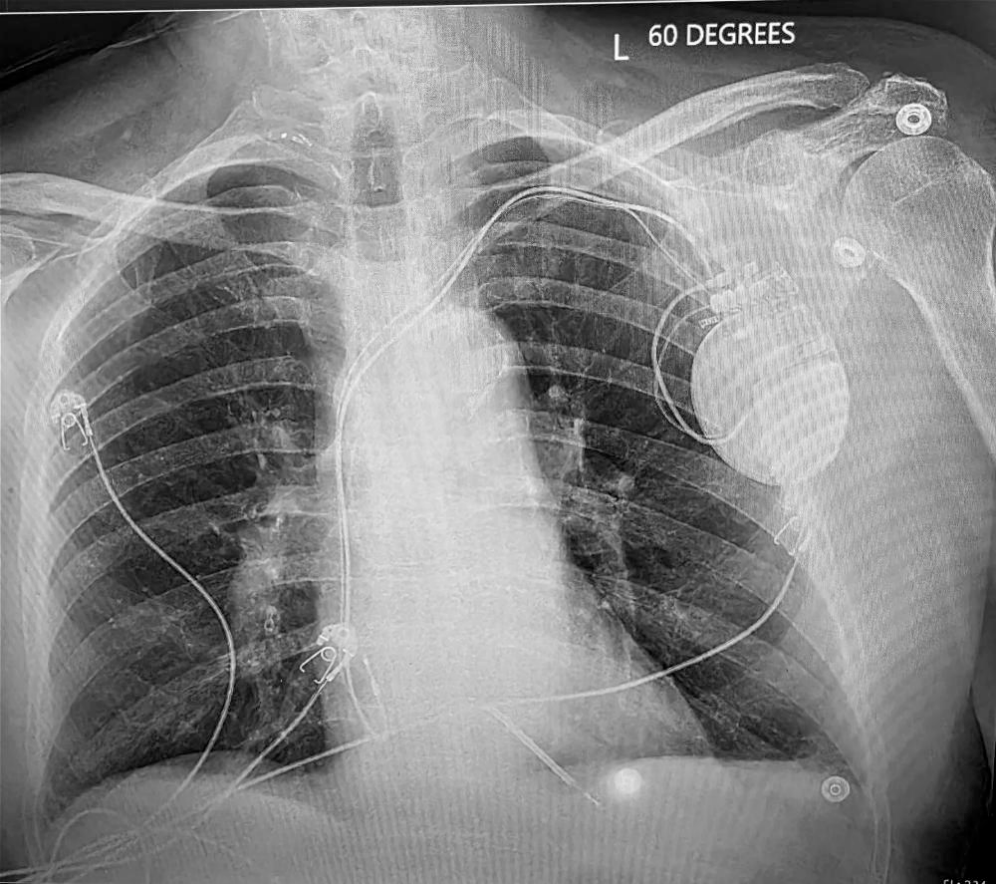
Post-implantable cardioverter-defibrillator replacement chest radiograph showing correctly positioned right atrial and right ventricular leads.
